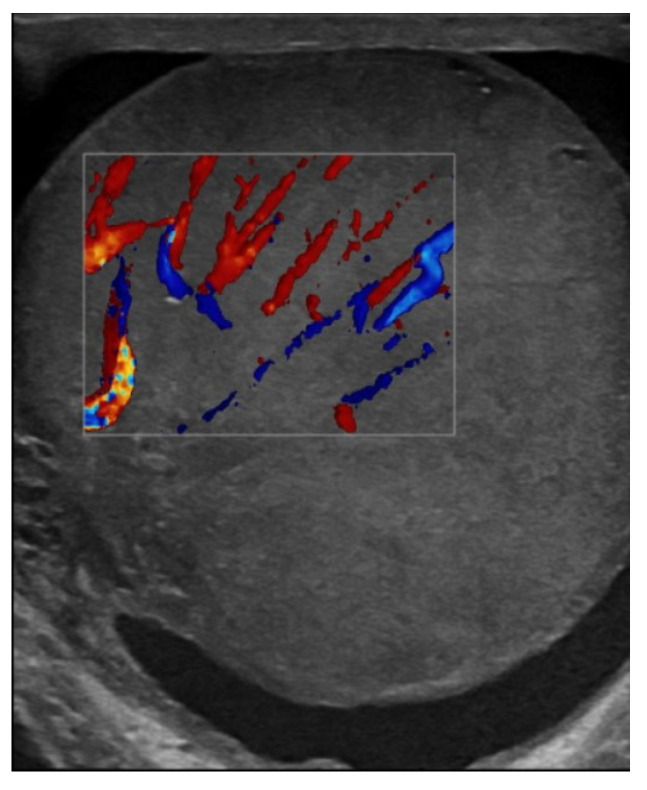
Vascular signal present in Doppler.
